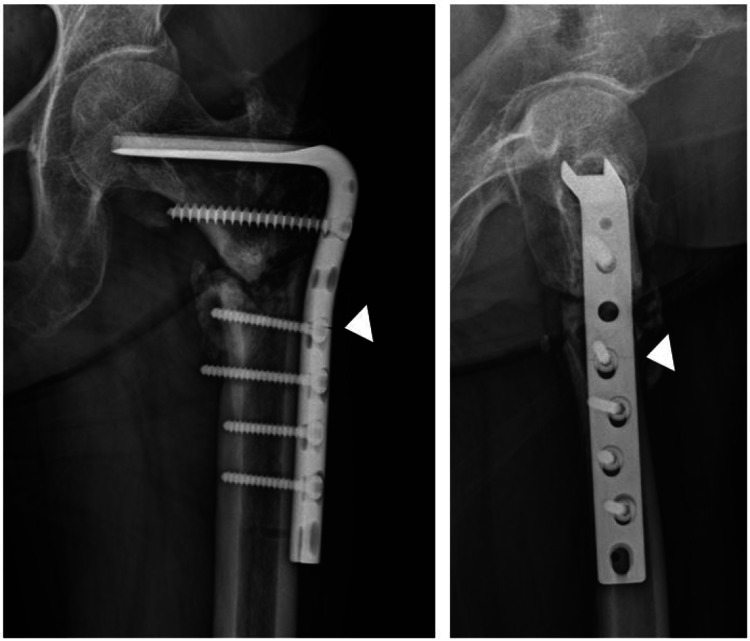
Simple radiographs 13 months after revision surgery shows breakage of plate (arrowhead).
